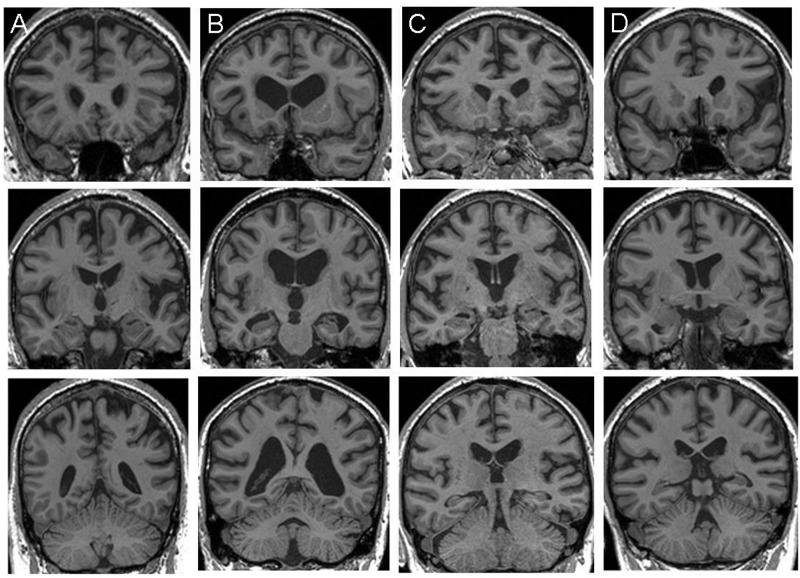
Representative coronal T1-weighted MR brain sections for individual patients (designated A to D) with C9ORF72-associated frontotemporal dementia (MRI was contraindicated in one case). Each column corresponds to a single patient; sections have been selected to capture the anterior frontal lobes and temporal poles (top row), anterior peri-Sylvian regions and medial temporal lobes (middle row), and posterior parietal lobes and cerebellum (bottom row). The left hemisphere is shown on the right in all sections.
